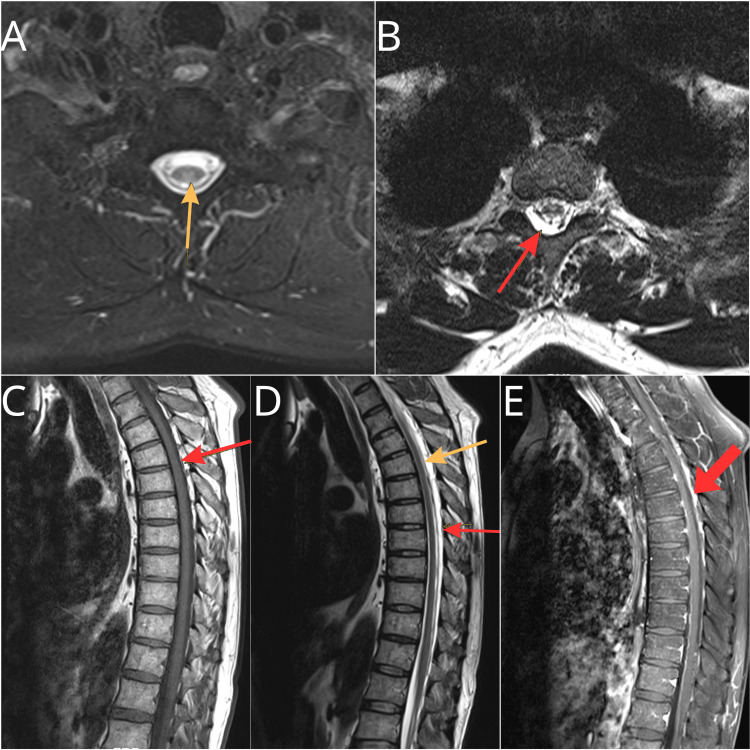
Magnetic resonance imaging of the thoracic spine Axial T2-weighted (T2W) image at T4 (A) and sagittal T2W image at T3–T8 (D) reveal hyperintensity of dorsal columns (yellow arrow). Axial T2W image at T6 (B) demonstrates a subdural fluid collection (red arrow). Sagittal T1-weighted (T1W) image (C) and T2W (D) reveal thickening of the dorsal dura mater (red arrow). Sagittal contrast-enhanced T1W image (E) shows marked enhancement of the thickened dorsal dura mater (bold red arrow).
